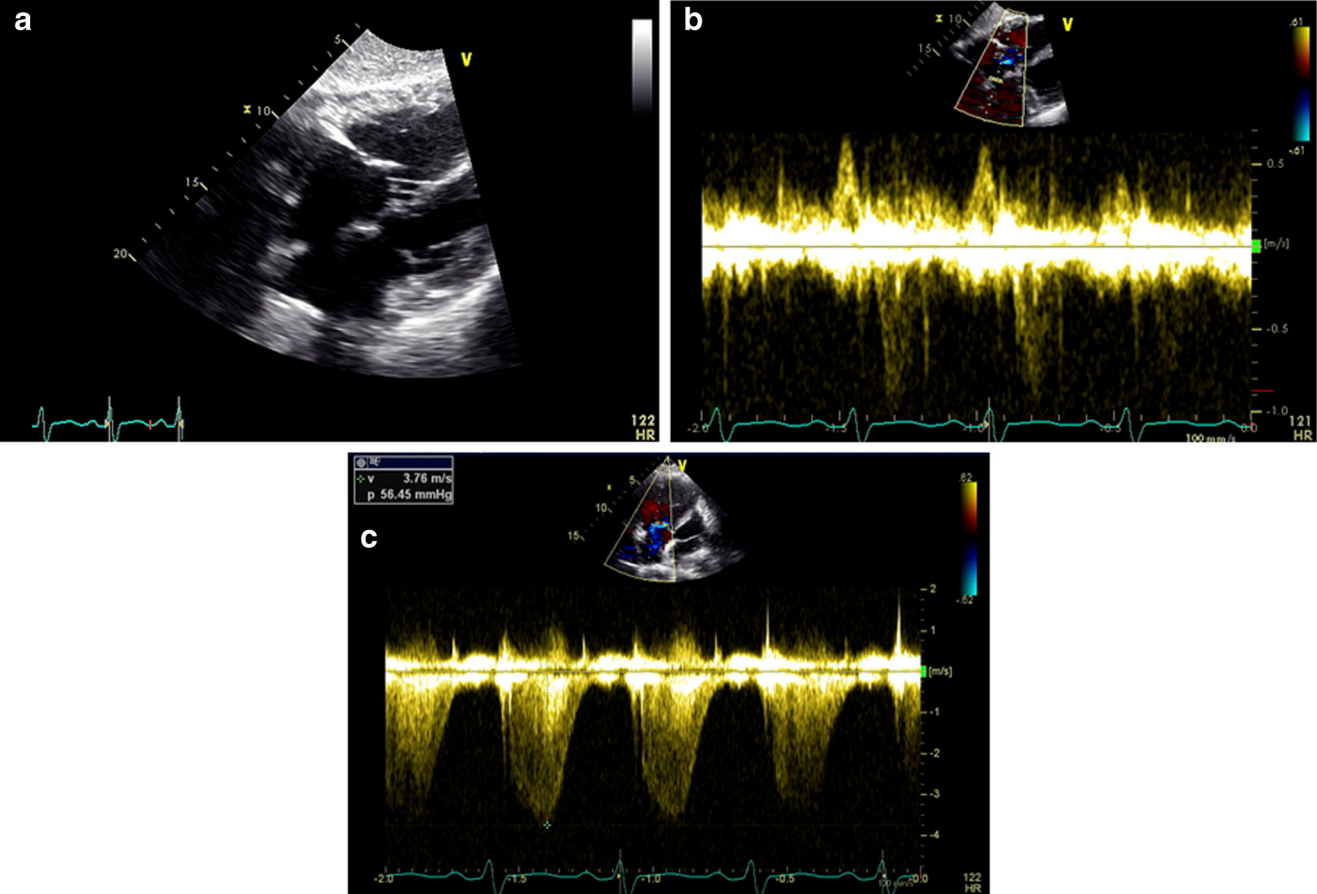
Incidental secundum atrial septal defect (ASD) measuring 1.9 × 1.5 cm seen in a pregnant patient who decompensated during delivery (a). Echo-Doppler revealed bidirectional shunting (b) and severely elevated peak right ventricular systolic pressure (RSVP), greater than 55 mm Hg (c). Patient was treated with intravenous diuretics and vasodilators. RVSP improved and patient was discharged home
